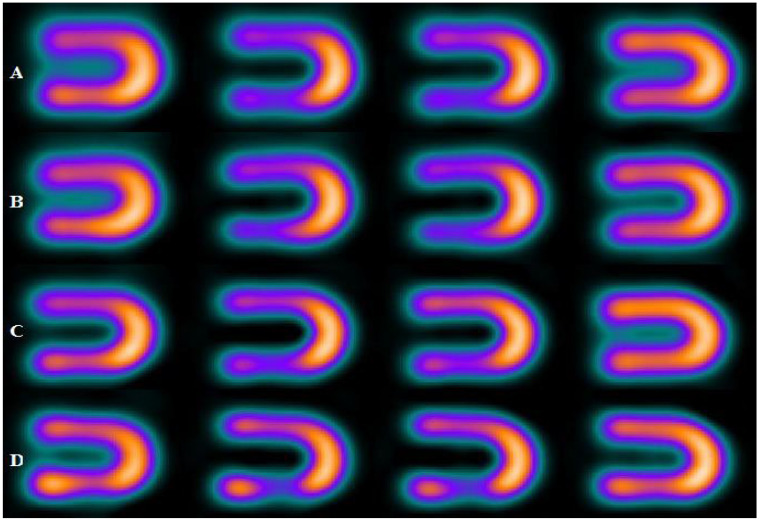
Defect in interior and inferior region of myocardial SPECT image using 20% energy window at four positions, where the rows (A, B, C, and D) at (the center, 5 cm, 10 cm, and 15 cm), respectively.
